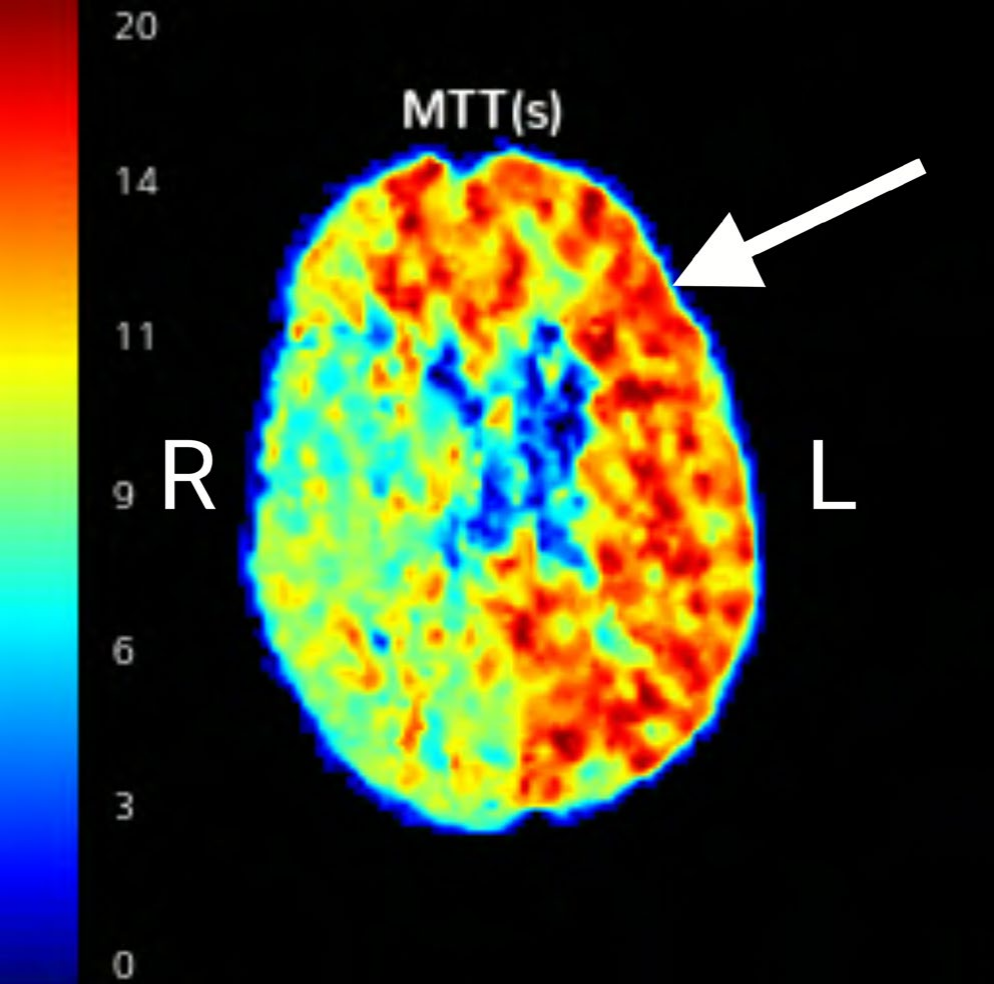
This computed tomography angiogram perfusion study shows increased mean transit time (MTT) in seconds on the left. At‐risk penumbra tissue in red (see arrow) involves the left (L) middle cerebral artery and bilateral anterior cerebral artery distribution involving the Circle of Willis and the retrograde ophthalmic artery. Normal flow is yellow‐green on the right (R). Penumbra is 12–14 s on the left or roughly 2.5 times longer than the contralateral normal side. When there is an arterial blockage to the brain, blood is slow to flow in and slow to wash out, so there is prolonged transit time. The left side of the brain is the abnormal side with prolonged transit time, and the right side is normal.
